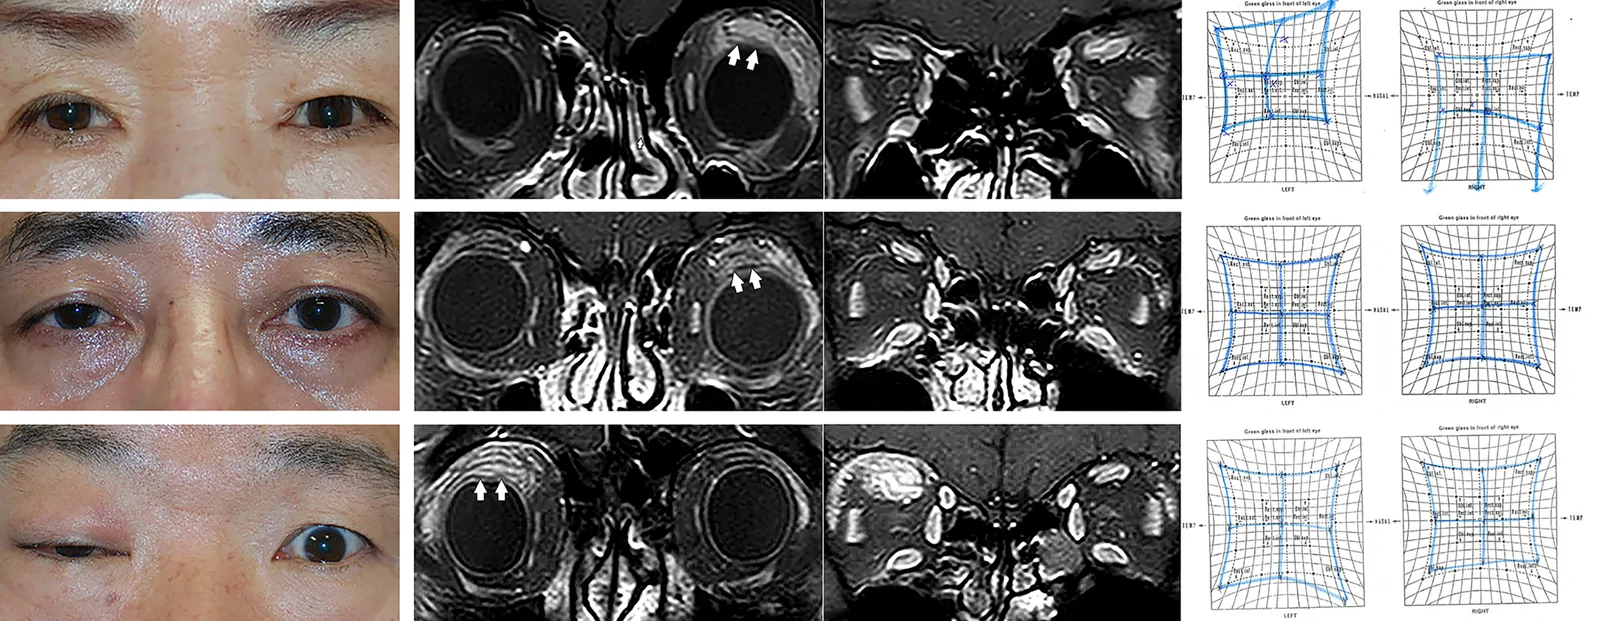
Representative cases with isolated superior rectus-levator complex (SR-LC) enlargement. The magnetic resonance images are sharpened coronal sections at the SR tendon (arrow) insertion site and muscle belly. (Top row) Thyroid eye disease manifested as upper lid retraction, tendon-sparing SR enlargement, and restricted infraduction of the left eye. (Middle row) Idiopathic orbital myositis (IOM) with upper lid retraction manifested as tendon-involving SR enlargement with minimal adjacent infiltration and restricted supraduction of the left eye. (Bottom row) IOM with upper lid ptosis manifested as tendon-involving SR enlargement with marked infiltration into the adjacent orbital wall and intermuscular septum, and restricted infraduction of the right eye.
